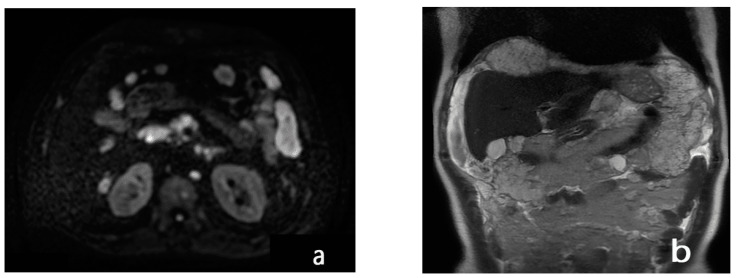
A 75-year-old man with a diagnosis of cecal adenocarcinoma. DWI b1000 axial (a) and T2w coronal (b) MR images of the upper abdomen reveal widespread nodular peritoneal carcinomatosis causing compression of the intra-abdominal organs, particularly at the level of the body of the gall bladder and cystic duct, accompanied by free fluid in perihepatic, perisplenic, and intestinal-loop locations.
